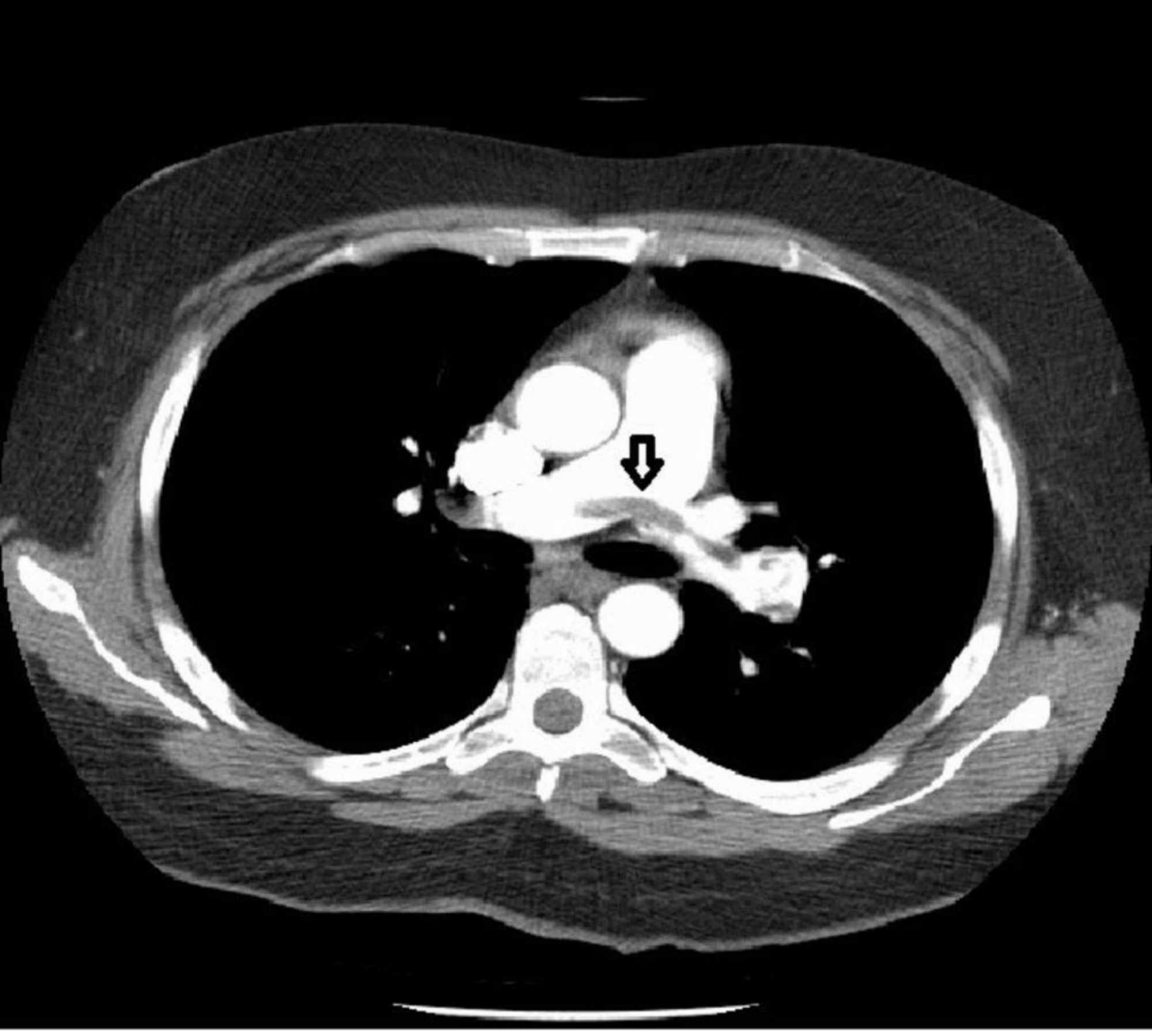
CT pulmonary angiogram showing saddle embolus in the main pulmonary artery
